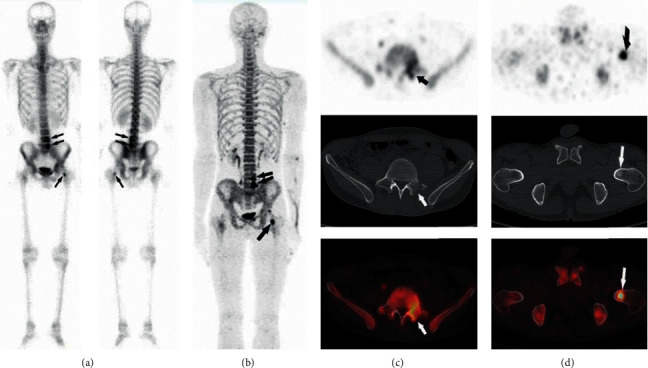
A 36-year-old man with NPC and confirmed as bone metastases by follow-up in the 5th lumbar spine, sacral spine, and left femoral neck. All modalities show clear evidence of metastasis: focal accumulation in planar ^99m^Tc-MDP bone scintigraphy (a) (arrowheads), tracer uptake in ^18^F-NaF PET (b) (arrows), and cortical destruction (c) and osteolysis in CT (d).
